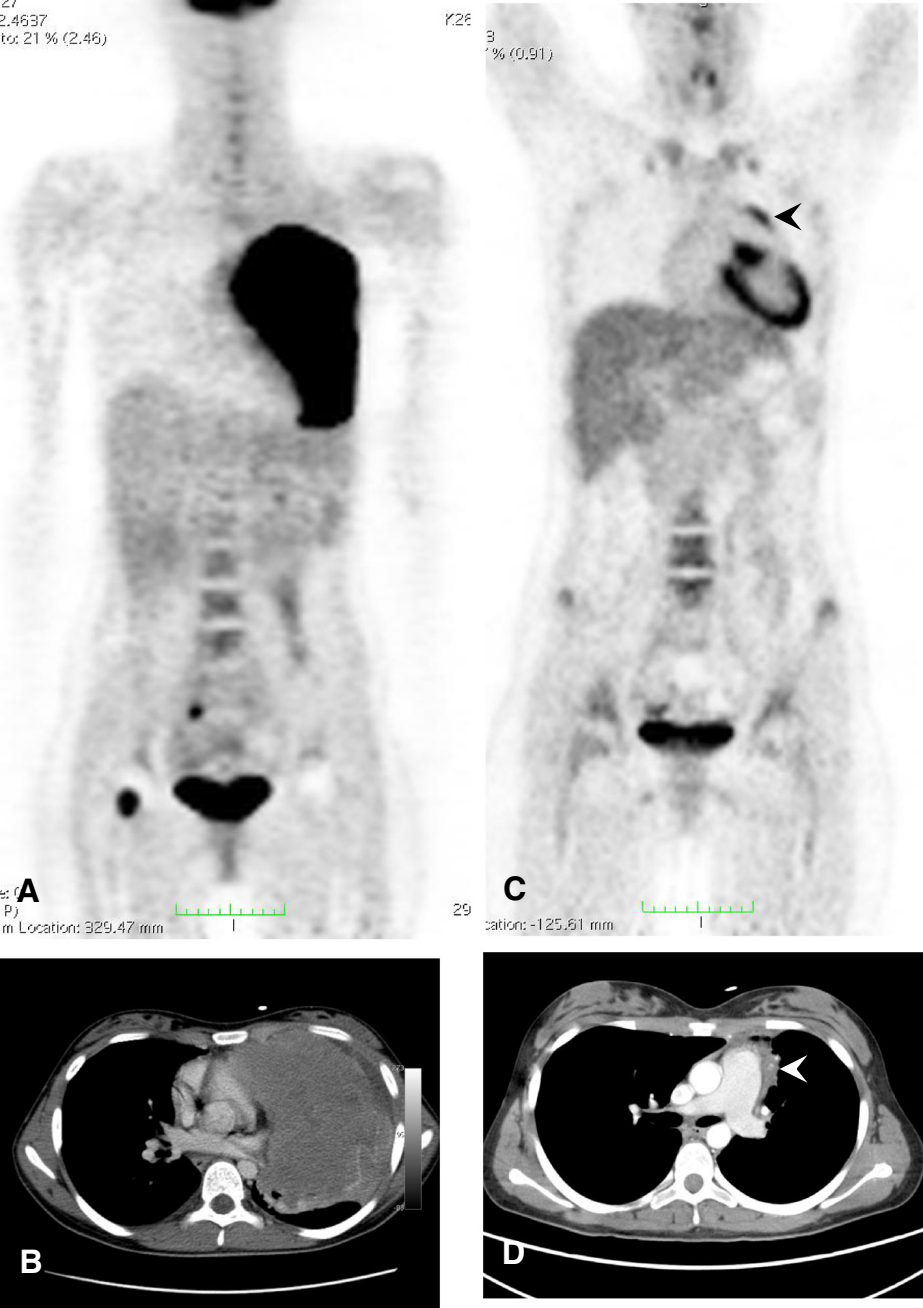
Sixteen-year-old girl suffering from a diffuse large B cell lymphoma (Table2, patient 5). The lymphoma was primary localized in the left lung and pleura (A, B). Initial SUVmax was 10.7 and decreased to 4.2 at interim (A and C; visual iPET-positive, *Δ*SUVmax 60.7%). Thus, PET approaches suggested residual disease at interim. CIM findings at interim showed massive reduction in size (arrow head, D) and disappearance of all other foci corresponding to a complete response unconfirmed. The patient is in ongoing remission (CIM, true-negative; visual iPET, false-positive; *Δ*SUVmax, false-positive).
